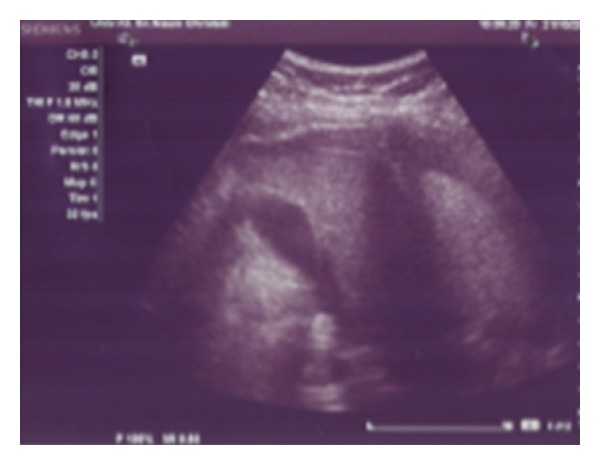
Abdominal ultrasound shows ascites without findings of liver cirrhosis or portal hypertension.
